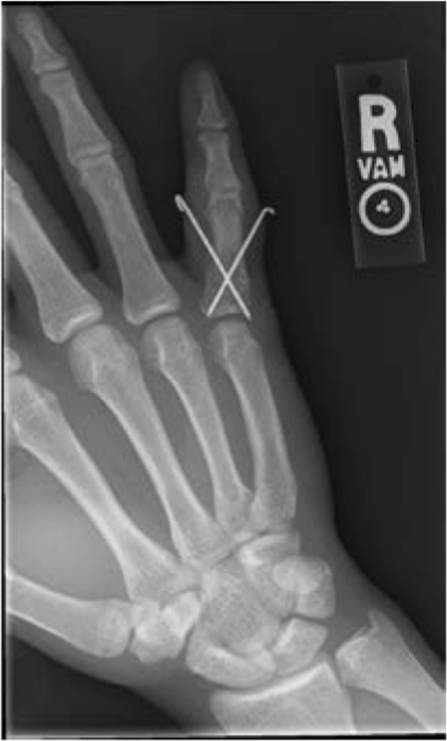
A PA radiograph of a small finger transverse proximal phalanx fracture with clinical malrotation which was treated with closed reduction and crossed K-wires
